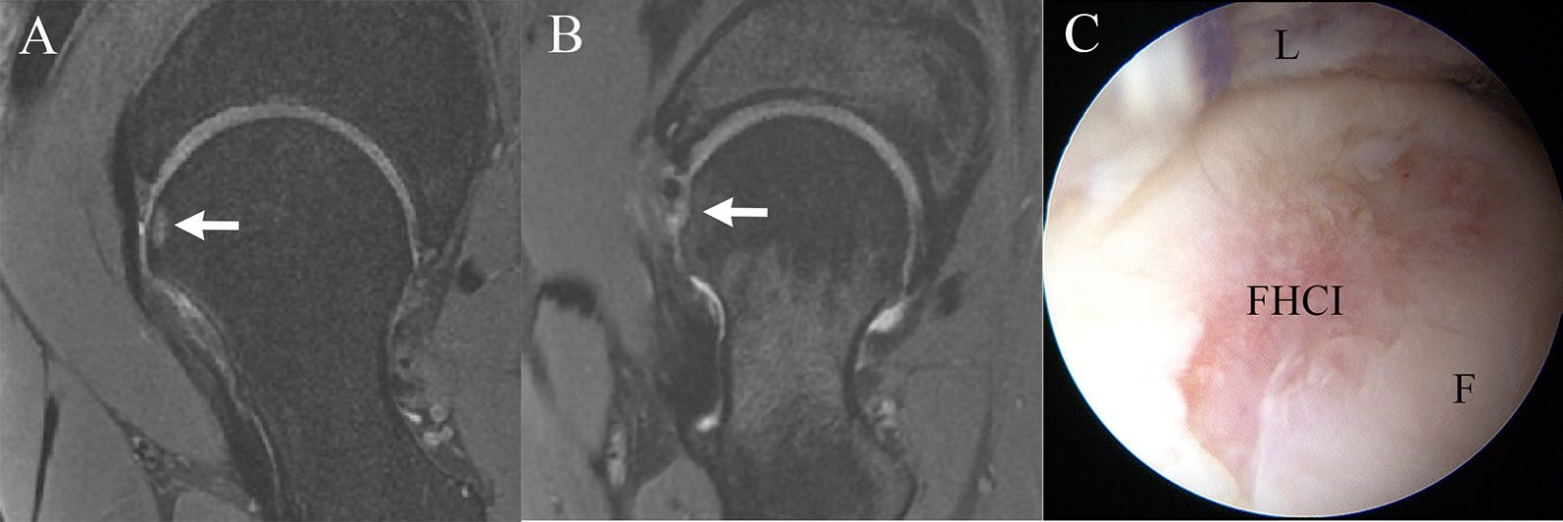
A Postoperative FHCI identified as concavity with a small area of hyperintensity of underlying bone observed on MRI. B Postoperative FHCI identified as a flattening of the head sphericity observed on MRI. C Postoperative FHCI observed in hip arthroscopy. White arrow, the area of postoperative femoral head cartilage injury. *FHCI* femoral head cartilage injury, *L* labrum, *F* femoral head
